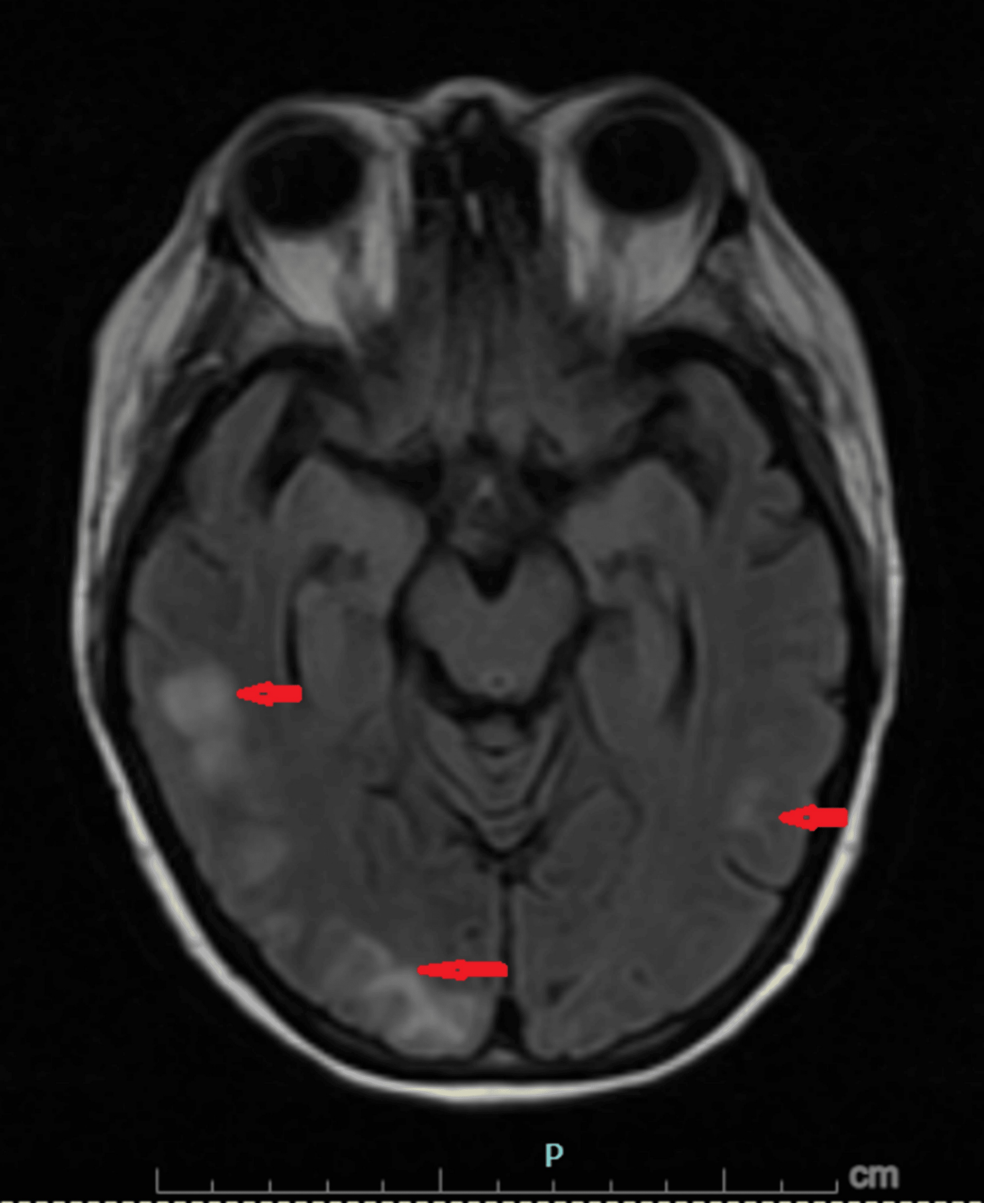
Posterior cerebral involvement on brain MRI Magnetic resonance imaging (MRI) of the brain with fluid-attenuated inversion recovery (FLAIR) sequences demonstrating subcortical hyperintensities (red arrows) involving the bilateral cerebral hemispheres with posterior predominance. There is no associated mass effect, diffusion restriction, or abnormal enhancement. These findings are characteristic of posterior reversible encephalopathy syndrome (PRES).
